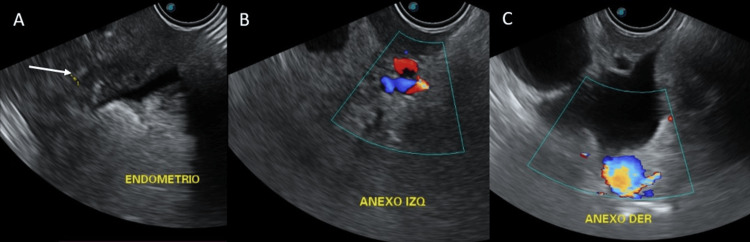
Transvaginal Ultrasound Findings (A) Thickened and echogenic endometrium (white arrow). (B) Left adnexa without suspicious findings on Doppler evaluation. (C) Right adnexa without suspicious findings on Doppler evaluation.
